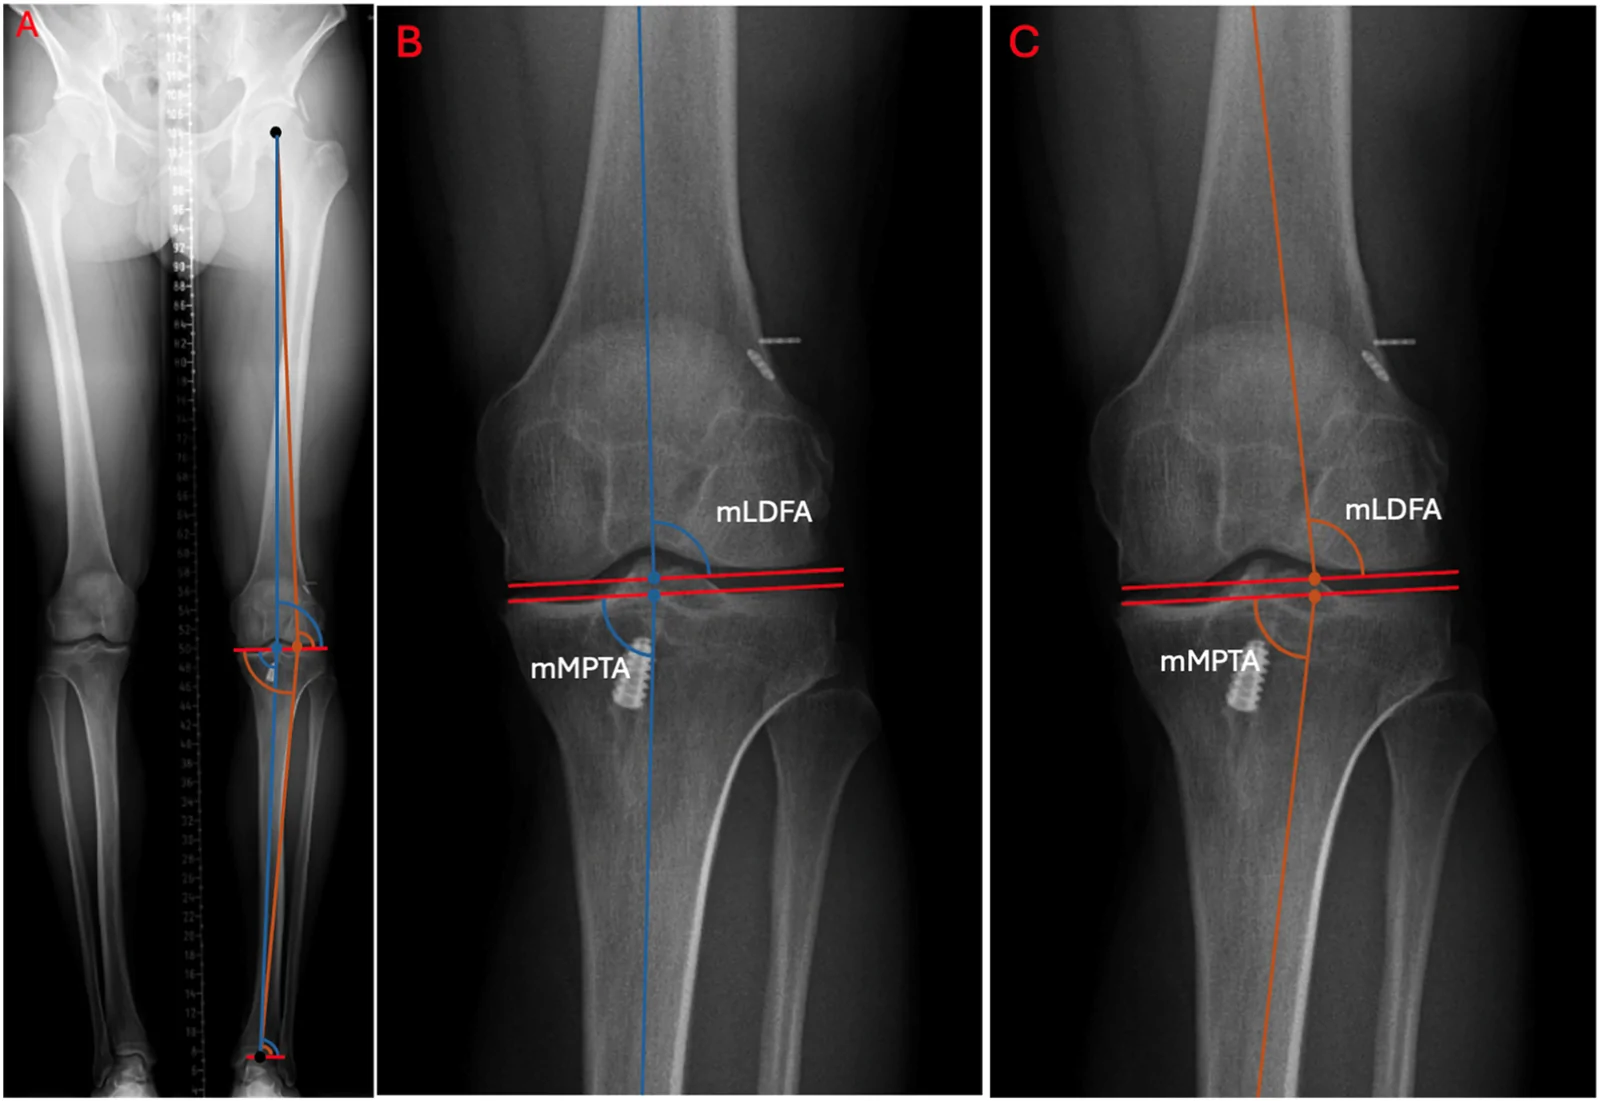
Lower extremity radiographs showing (A) the mechanical axis of the femur and the mechanical axis of the tibia from the center of the knee point (blue lines) with their component mLDFA & mMPTA angles (blue angles). The mechanical axis of the femur and the mechanical axis of the tibia from a 62.5% lateral weight-bearing point (orange lines) with their component mLDFA & mMPTA angles (orange angles). (B) Knee radiograph showing center of the knee analysis with the component angles mLDFA and mMPTA shown (blue lines) being measured from the center of the knee. (C) Knee radiograph showing lateral weight-bearing point analysis with the component angles mLDFA and mMPTA shown (orange lines) being measured from a 62.5% lateral weight-bearing point
